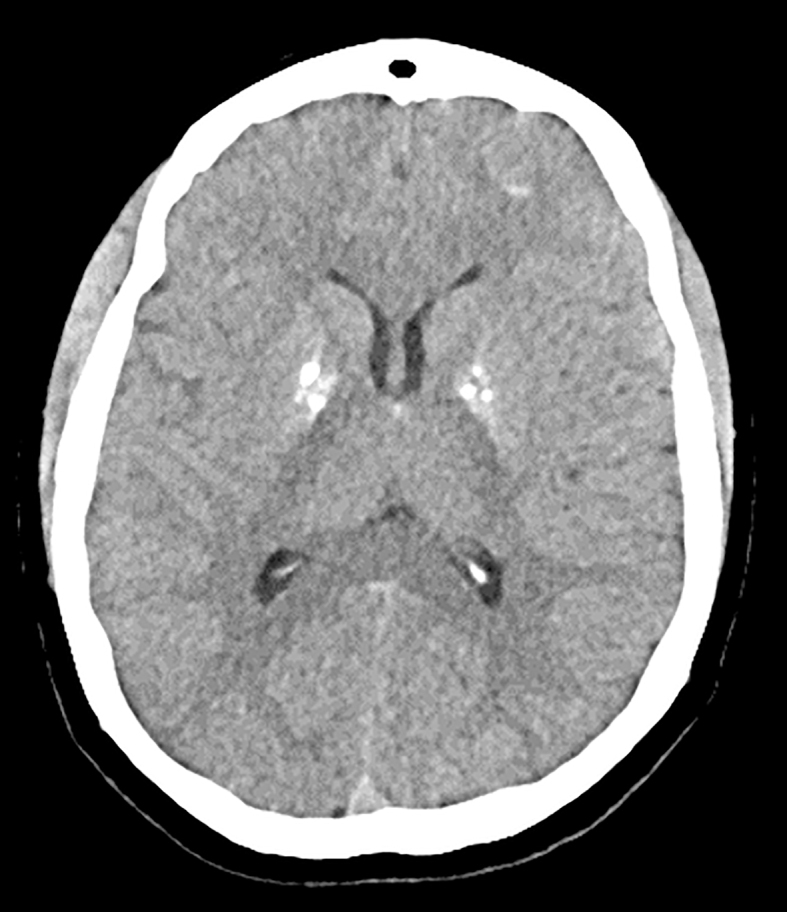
An axial non-contrast CT brain that shows bilateral punctate calcification of the globus palladi.
